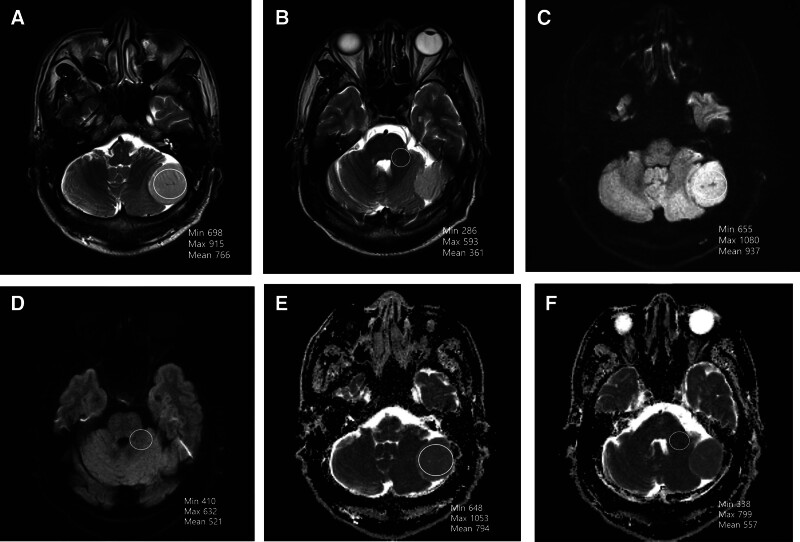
A 53-year-old woman with left cerebellar (soft meningioma). (A) Tumor ROI on T2-weighted MR sequence. (B) ROI in the middle cerebellar peduncle on T2-weighted MR sequence. The TCTI ratio = intensity value for ROI within tumor/intensity value for ROI within middle cerebellar peduncle = 698/286 = 2.44. (C) Tumor ROI on DWI MR sequence. (D) ROI in the middle cerebellar peduncle on DWI MR sequence. The TCTI ratio = intensity value for ROI within tumor/intensity value for ROI within middle cerebellar peduncle = 655/410 = 1.695. (E) Tumor ROI on ADC MR sequence. (F) ROI in the middle cerebellar peduncle on ADC MR sequence. The TCTI ratio = intensity value for ROI within tumor/intensity value for ROI within middle cerebellar peduncle = 648/338 = 1.670. ADC = apparent diffusion coefficient, DWI = diffusion-weighted imaging, ROI = region of interest, TCTI = tumor-to-cerebellar peduncle T2-weighted imaging intensity.
